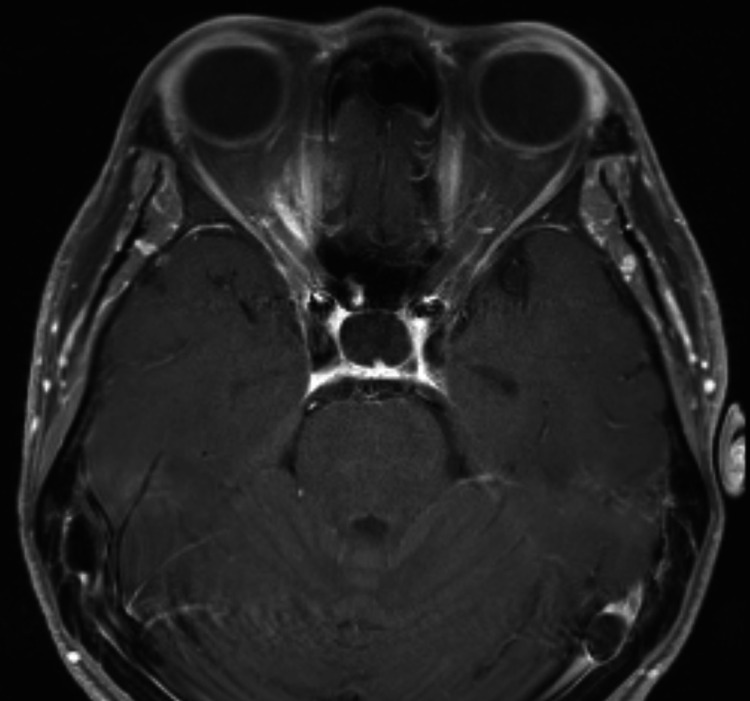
MRI nine months post-radiosurgery. Axial T1 contrast-enhanced MRI done nine months after GKRS demonstrating no significant change in the size of the tumor although it had a more diffuse appearance. GKRS: Gamma Knife radiosurgery; MRI: magnetic resonance imaging
